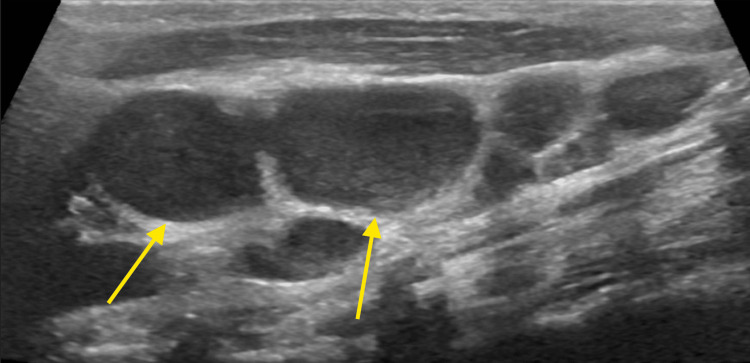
Neck ultrasonography showing right-sided lymphadenopathy (arrows)
